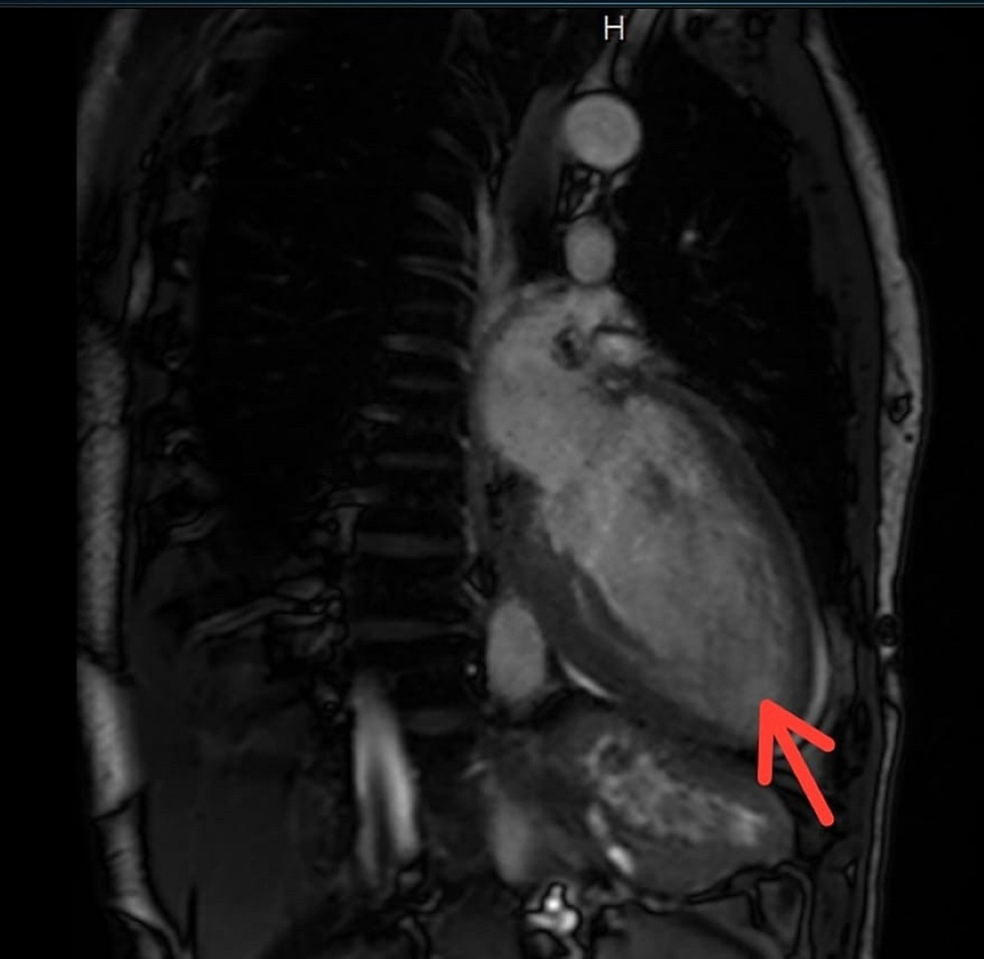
Cardiac MRI revealed a severely dilated left ventricle with marked noncompaction and prominent trabeculations in the region of the true apex The red arrow highlights the area of non-compacted apical left ventricular myocardium.
